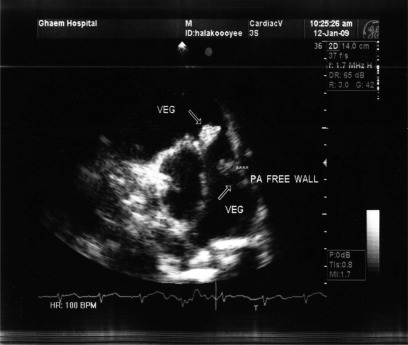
Parasternal short axis view revealed two vegetations on pulmonic valve and PA free wall
